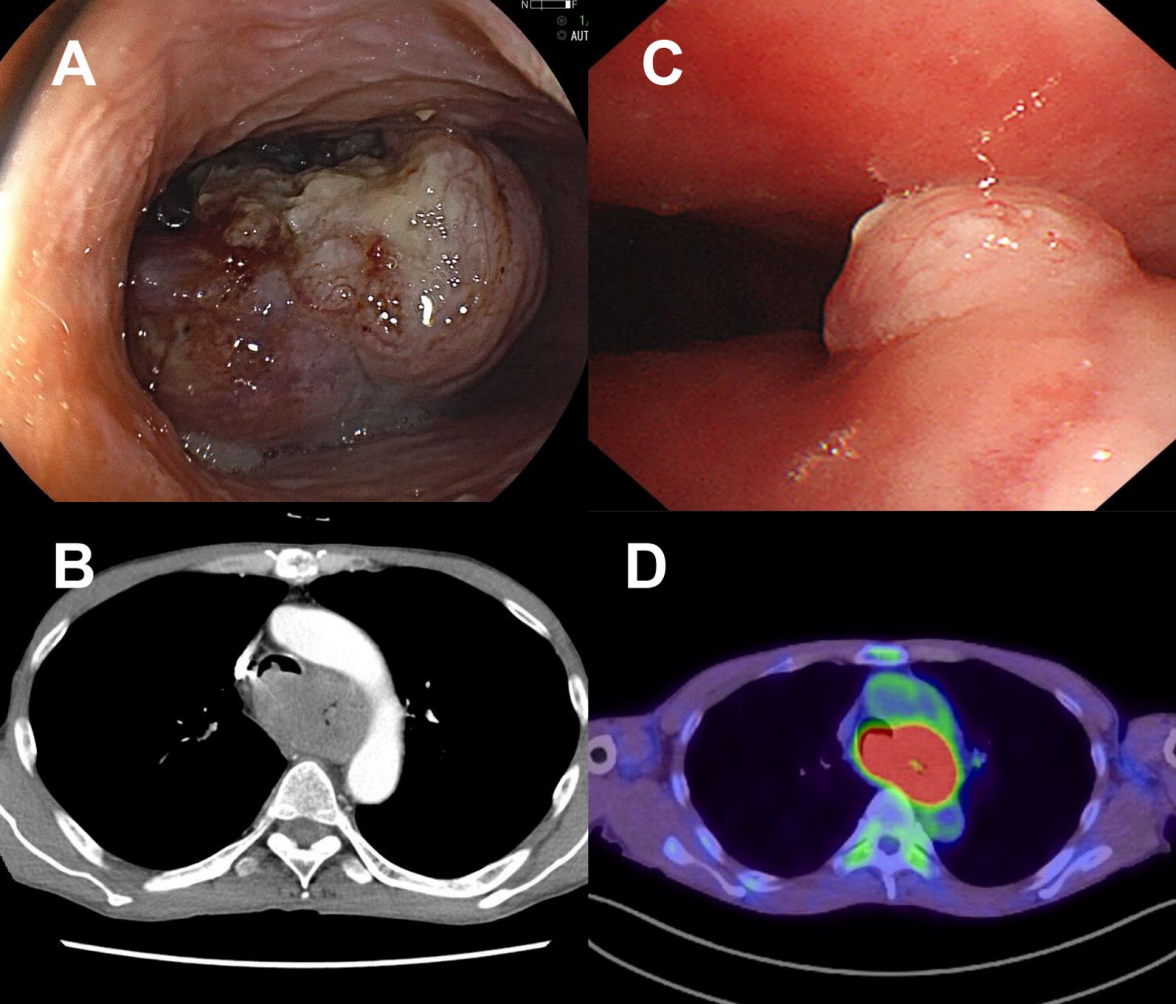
Initial medical examinations before treatment. A Esophagogastroduodenoscopy (EGD) image. A full circumferential type 3 tumor was detected in the upper thoracic esophagus. The scope was impassable. B Contrast-enhanced CT. A lumen-filling tumor with invasion of the tracheal bifurcation in the thoracic esophagus was shown. C Bronchoscopy. Partial tumor exposure near the tracheal bifurcation was indicated. D FDG PET-CT. There was an abnormal accumulation over a wide area of the thoracic esophagus, which was united with lymph nodes. There were no obvious distant metastases
